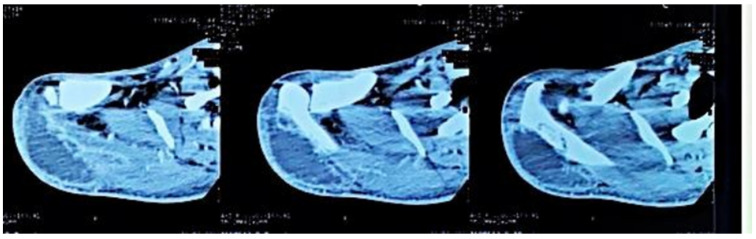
Axial sections of the shoulder CT scan, showing the deltoid collection.
